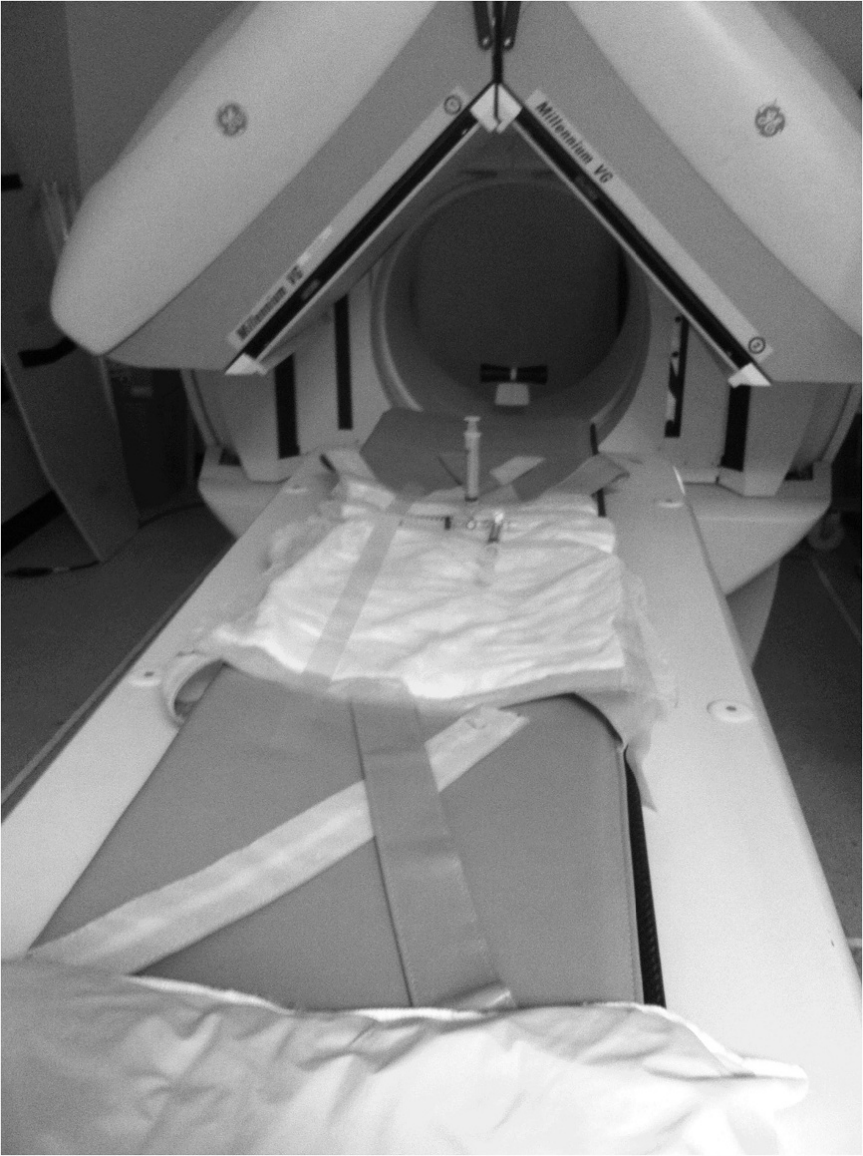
Experimental setup. Syringe-set at 1 cc in the A-SPECT camera just before rotating the detector to the start position.
